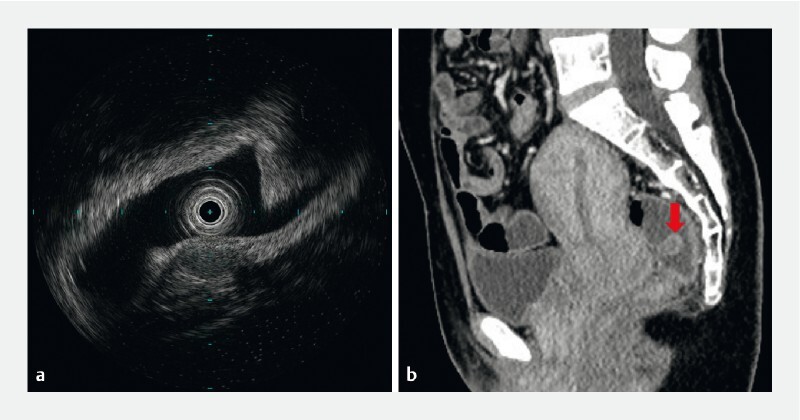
Endoscopic ultrasound suggested that the lesion originated from the submucosal layer, and that it locally involved the muscularis propria.
